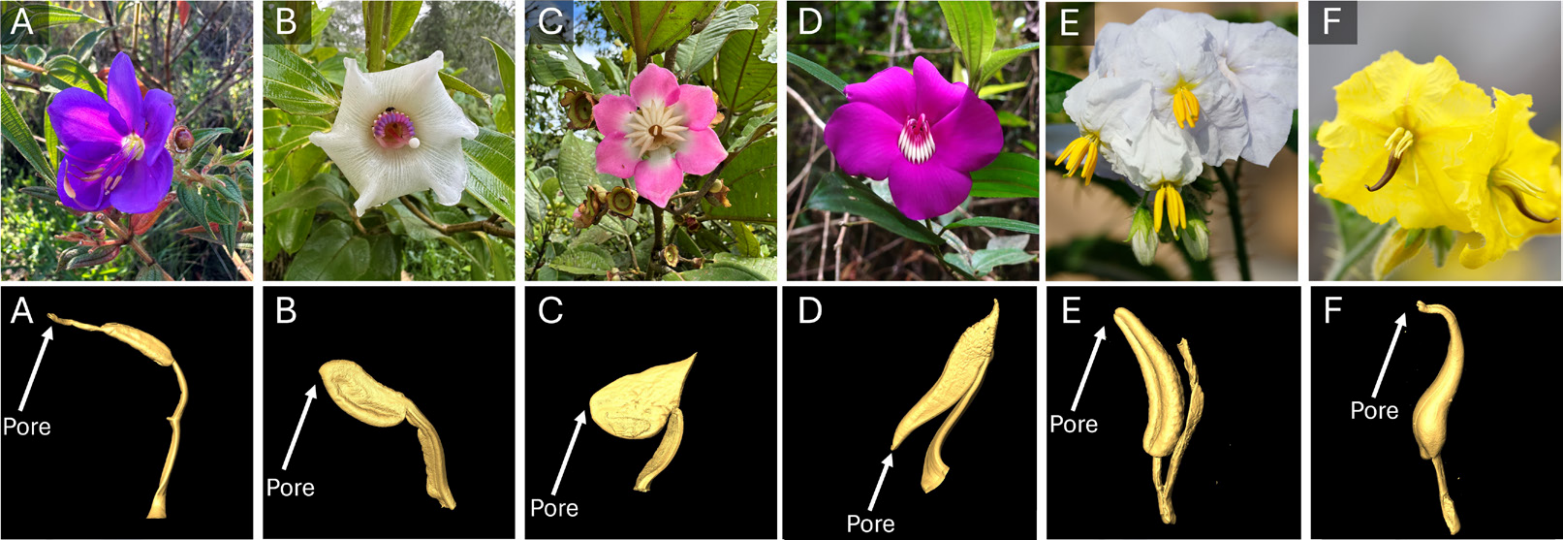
Buzz-pollinated flowers exhibit a wide range of diversities. Buzz-pollinated flowers and their stamens come in a tremendous diversity of shapes and sizes. The role of these disparate morphologies is still poorly understood, with hypotheses ranging from pollination niche partitioning (i.e. specialization on different buzzing bees) to pollen dosing (limiting pollen release) to differential pollen placement on bees and beyond. This figure provides a few examples of the diversity seen in buzz-pollinated flowers of two of the largest radiations of buzz-pollinated flowers, the *Melastomataceae* plant family (>5800 spp.) and the genus *Solanum* (>1200 spp.), with corresponding CT scans of their stamens shown in the lower panels. The species shown, from left to right, are (A) *Rhynchanthera grandiflora* (Aubl.) DC., (B) *Blakea involvens* Markgr., (C) *Blakea* sp. nov*.*, (D) *Meriania speciosa* (Bonpl.) Naudin, (E) *Solanum sisymbriifolium* Lam. (study species of Alvord *et al*., 2025, photo credit Mario Vallejo-Marín), and (F) *Solanum rostratum* Dunal (photo credit Mario Vallejo-Marín). Other notable morphologies not shown in this figure include *Senna* which utilize curved petals to ricochet pollen onto buzzing visitors and *Pedicularis* species which have tubular petals, rather than anthers, which restrict pollen release to a narrow pore that must be buzzed to extract pollen.
